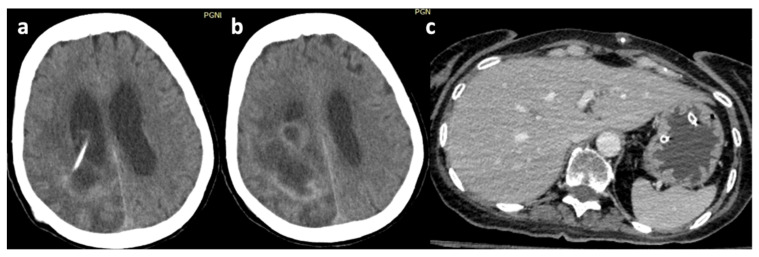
Axial head CT image showing: (a). infection of the brain parenchyma adjacent to the ventricular catheter and (b). an organized abscess cavity in the brain parenchyma. (c). Axial abdominal CT image demonstrating an abdominal abscess surrounding the tip of the peritoneal catheter.
